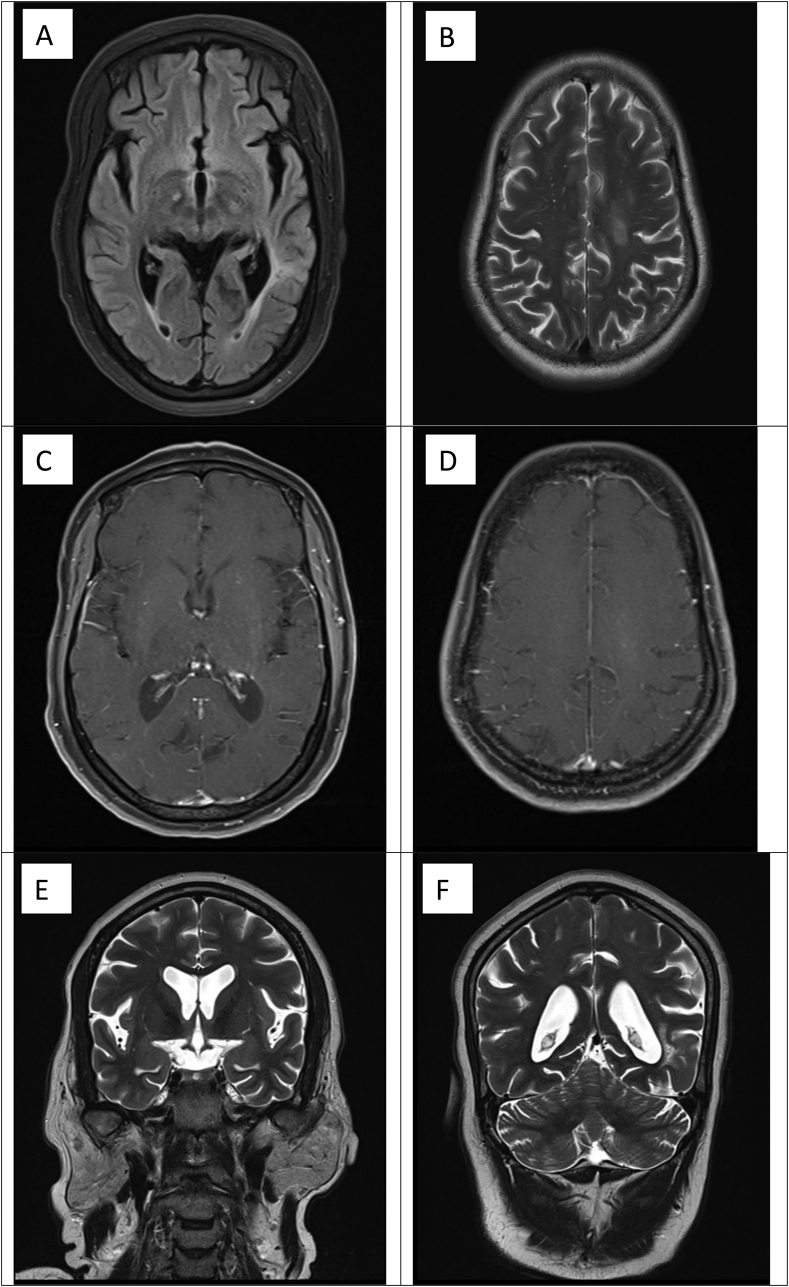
Brain magnetic resonance imaging (MRI) findings (Follow up). Confluent T2 and FLAIR hyperintensity in left centrum semioval, left posterior temporal, parietal deep and periventricular white matter. Faint enhancement in post-contrast. Non-enhancing, FLAIR hyperintense white matter foci in right frontal, parietal and occipital lobes and right & left sub lentiform internal capsule. No new lesions were noted in the follow-up study. Abbreviations: FLAIR, Fluid attenuated inversion recovery.
